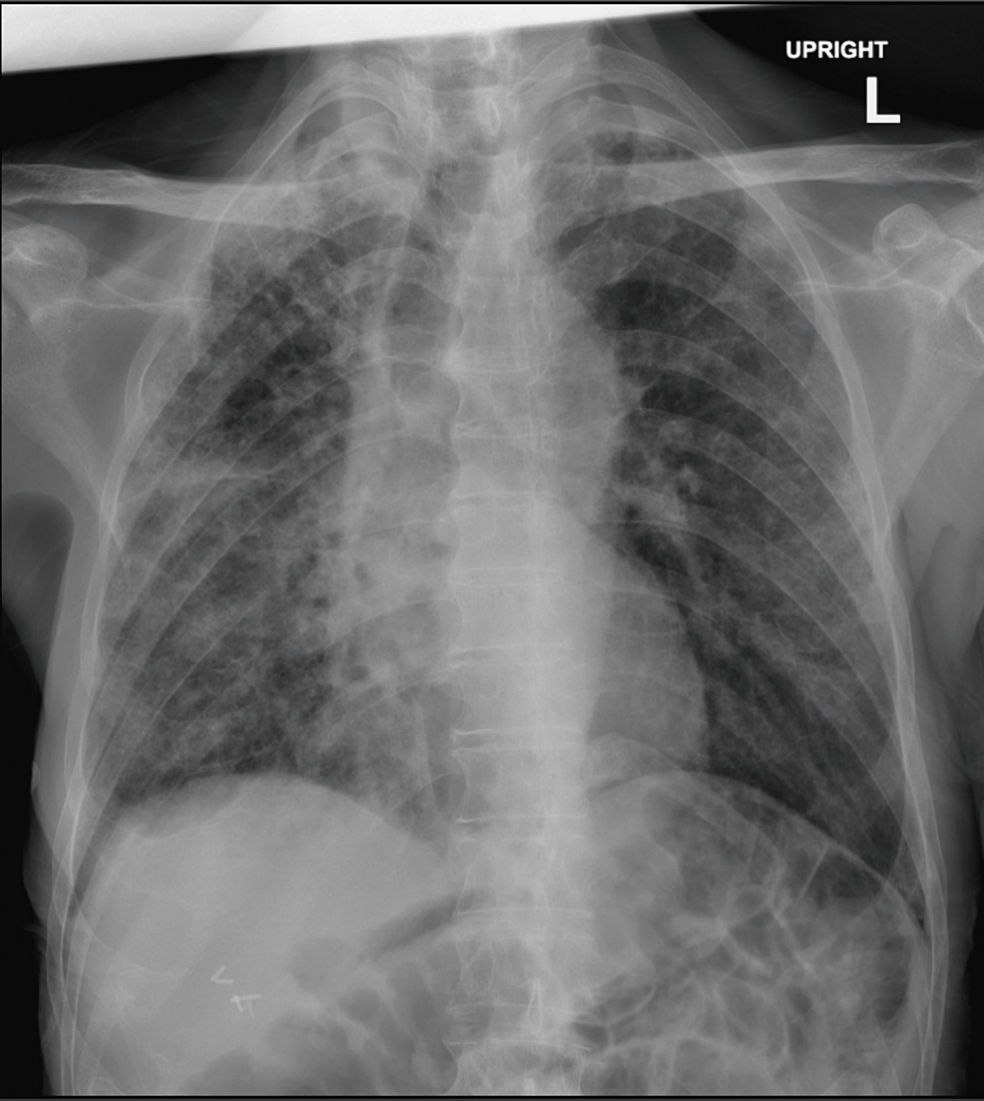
Chest X-ray performed at admission The image shows reticular opacity in the bilateral lung field.
